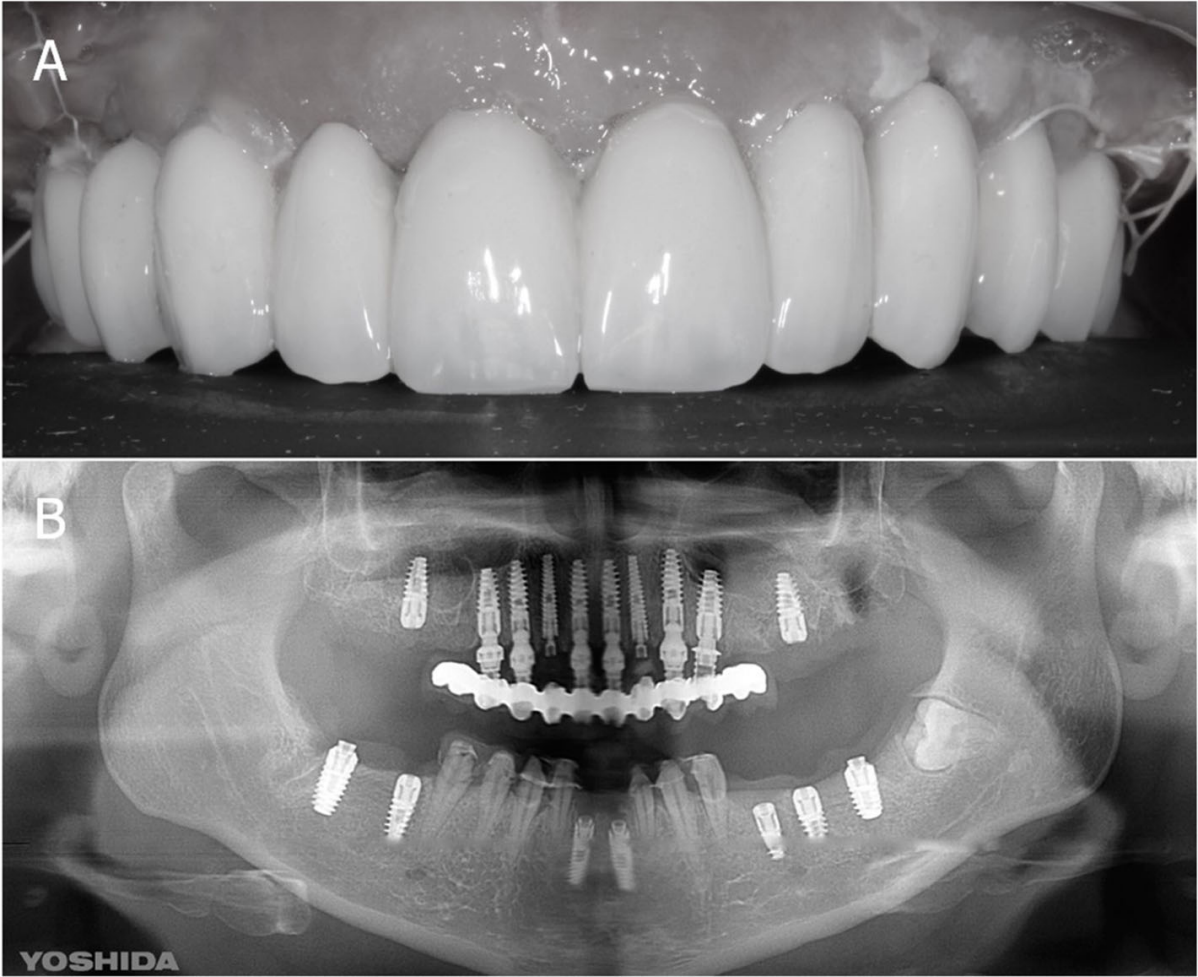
Immediate loading and panoramic control radiograph. a Temporary Prosthesis in position 3 days after surgery. b Post-operative situation: panoramic radiograph. During surgery, one more mandibular tooth had to be extracted and replaced by an implant
